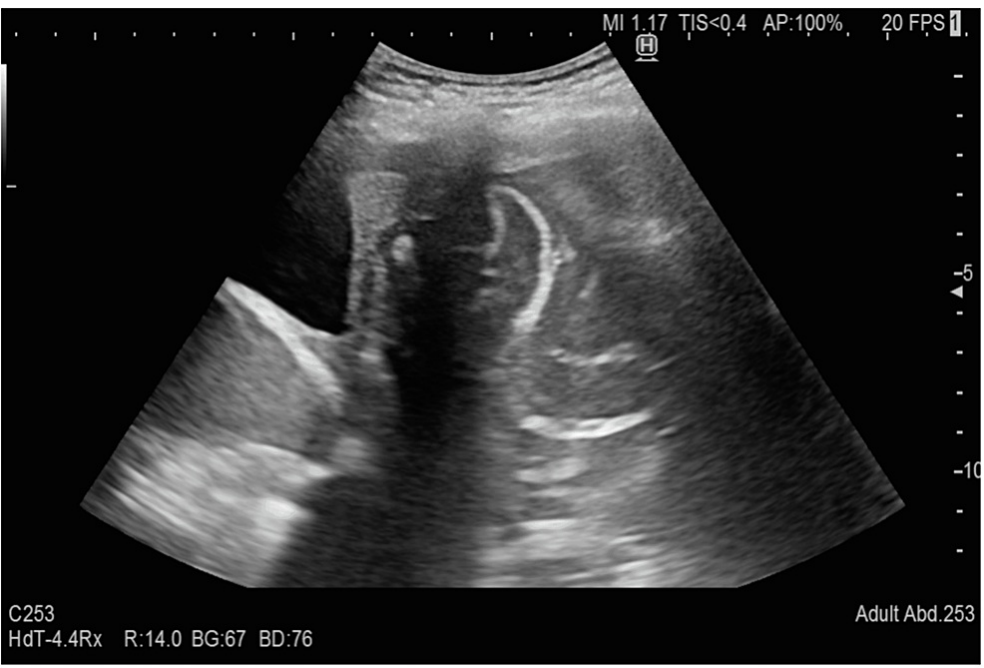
Lung ultrasound of the left lower posterior zone (PLAPS point) The figure demonstrates a complicated cyst.
